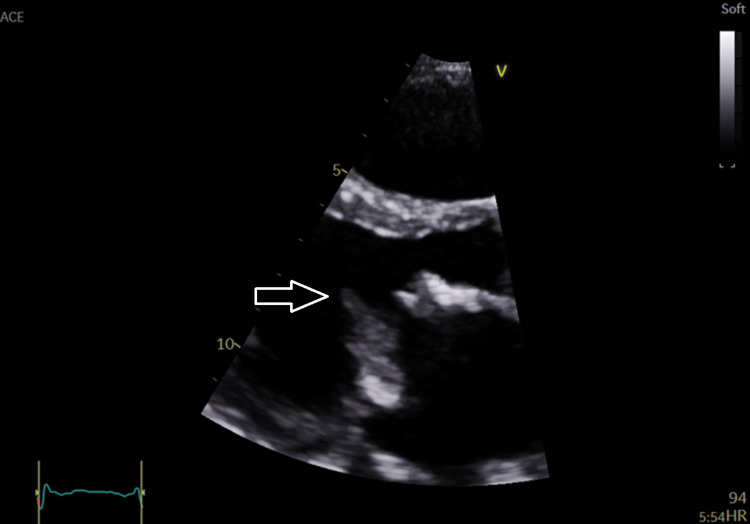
Shows PLAX view of prosthetic valve vegetations (white arrow) PLAX: parasternal long-axis
